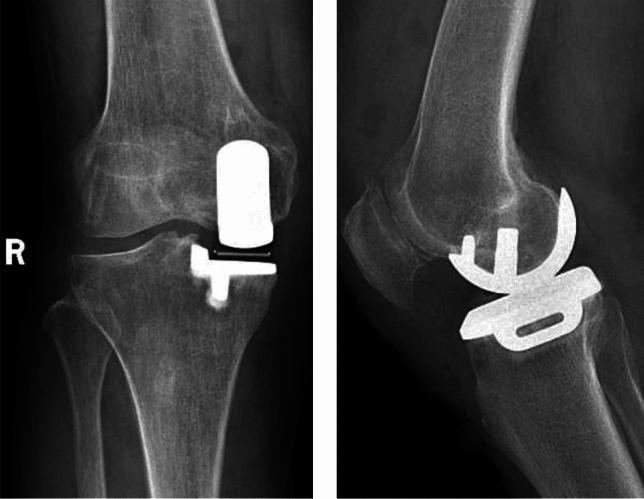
Radiographs of the anterior and lateral position of the knee joint showing changes after single condylar replacement of the right knee joint. The position of the prosthesis is satisfactory
